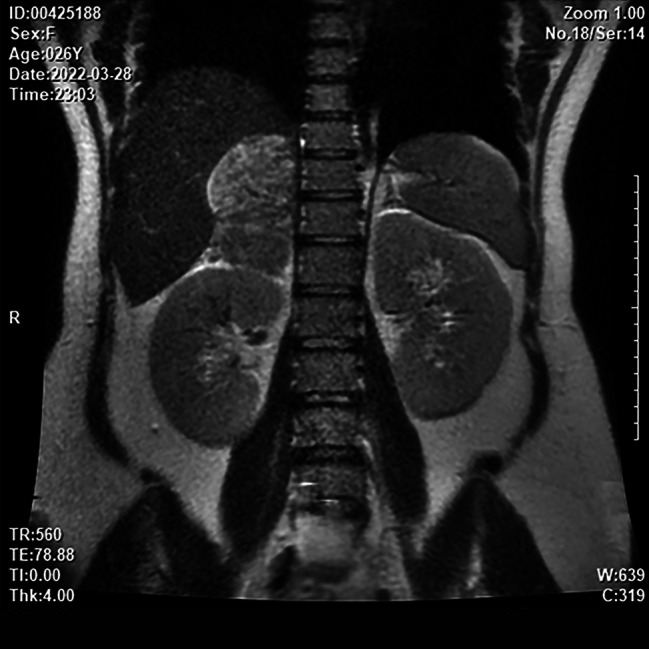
MRI of the urinary system showed a mass in the right adrenal gland with necrosis and hemorrhage
